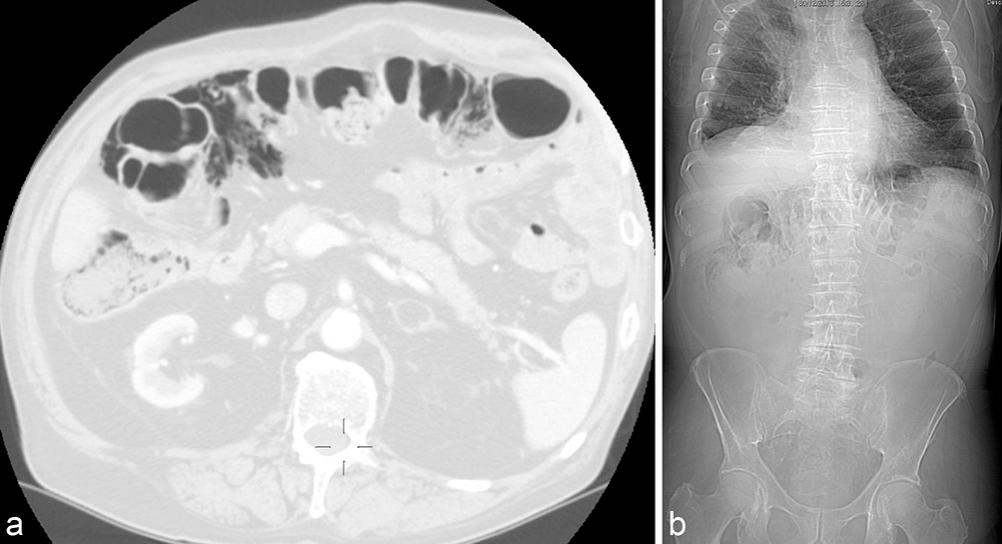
Case 3. An 80-year-old male receiving chemotherapy for lung adenocarcinoma and corticosteroids for chronic obstructive pulmonary disease-related dyspnoea. Chest CT; axial image, lung window. (a) Incidental finding of a large amount of gas in the adipose tissue along the caecum, and ascending and transverse colon; minimal intramural gas is confined to the caecum. The wall thickness is normal and haustra are regular. (b) Abdominal plain film performed a week later: persistence of a conspicuous amount of extravisceral gas along the caecum, right and transverse colon; no evidence of visceral distension.
